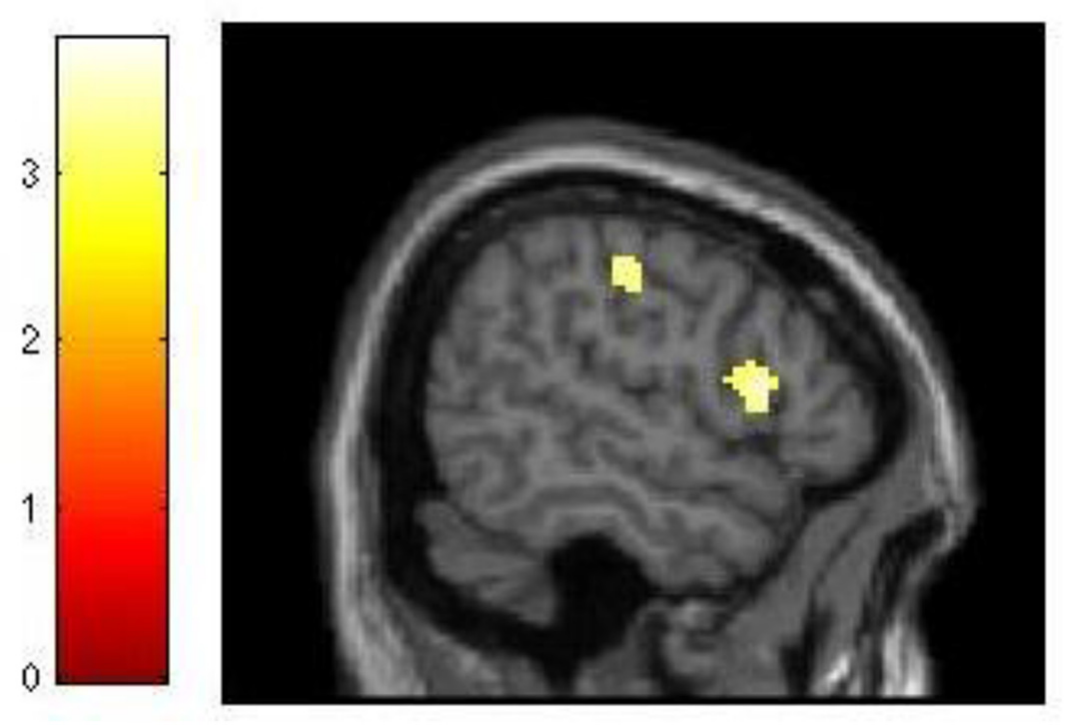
Relationship between training-related improvement in 3-back and brain activation in the maintenance phase of dMTS. Activation in right lateral PFC and motor cortex covaried with degree of training-related improvement in 3-back. SPM rendered into standard stereotactic space and superimposed on saggital MRI in standard space. Bar represents the corresponding *T*-score. dMTS, delayed Matching-to-Sample Task.
